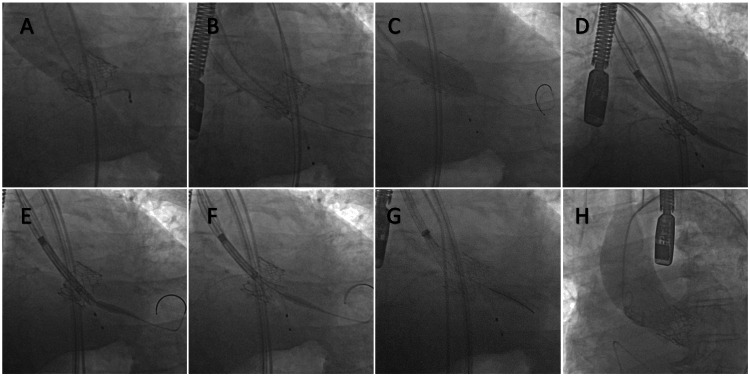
TEE- and fluoroscopy-guided Evolut-in-S3 ViV TAVR. TEE: Transesophageal echocardiography; TAVR: Transaortic valve replacement; ViV: Valve-in-valve; S3: Edwards Sapiens 3 pericardial tissue valve; Evolut: Medtronic Evolut FX self-expanding valve
